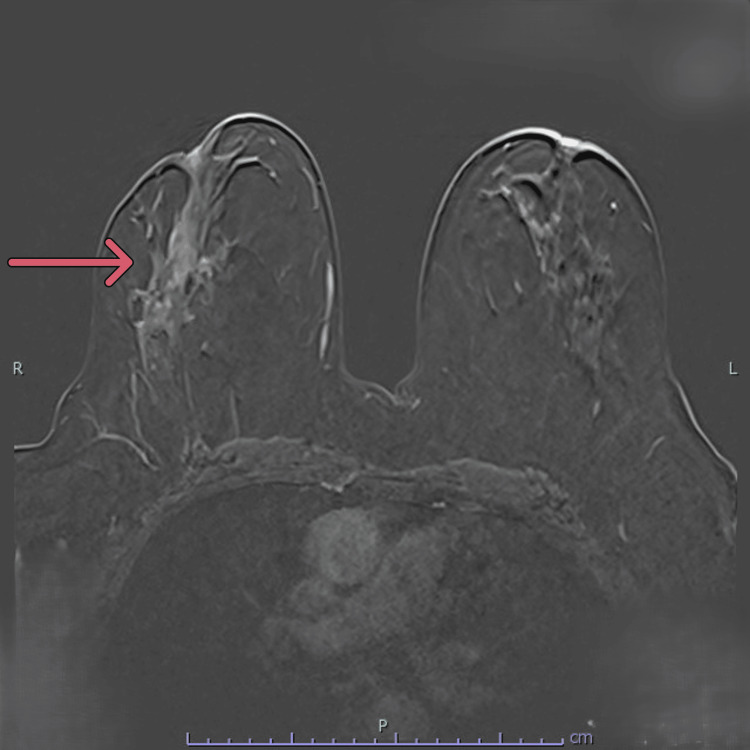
Breast magnetic resonance imaging (MRI). T1 post-contrast subtracted MRI image showing a segmental area of non-mass enhancement within normal parenchyma, highly suspicious for malignancy (the arrow indicates the area of interest).
